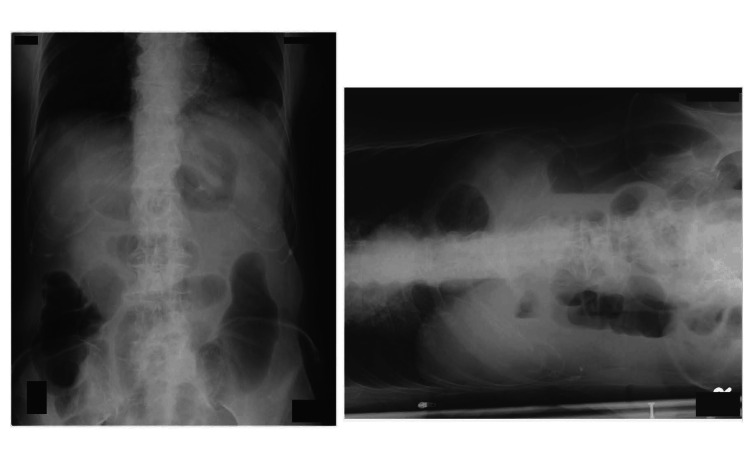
Bowel gas on X-ray Supine (left) and side-lying (right) abdominal radiographs. The side-lying position was used instead of the upright position to evaluate for air-fluid levels because the patient was unable to stand.
